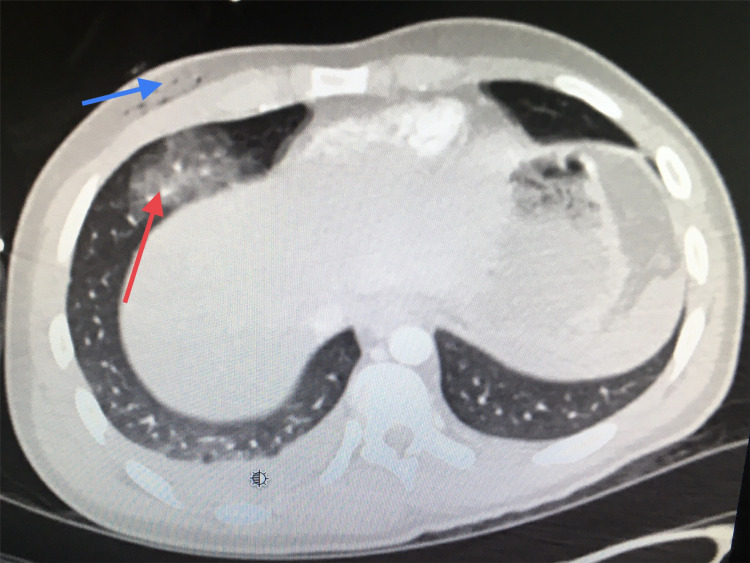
CT scan of the chest Red arrow shows lung laceration secondary to gunshot injury, and blue arrow shows subcutaneous air where the bullet traveled.
